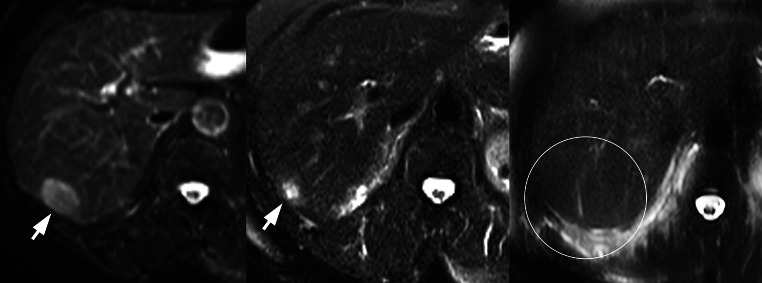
Radiographic response of a treated liver metastasis. The patient had a diagnosis of metastatic non-small cell lung cancer with evidence for a liver metastasis in segment 6. The *left panel* shows the pretreatment T2 fat-saturated image with the lesion noted by the *arrow*. The *central panel* shows the treated lesion approximately 6 weeks following radiation (35 Gy). The *right panel* shows the lesion approximately 6 months following radiation, with no evidence of residual tumor
